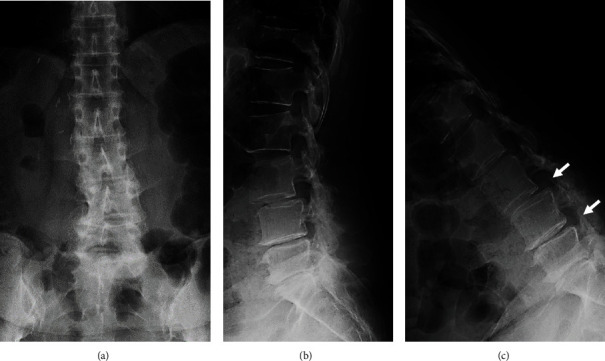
Preoperative lumbar spinal radiographs of a 49-year-old woman with degenerative lumbar disease. (a) anteroposterior view, (b) lateral view, and (c) lateral view (flexion position). Arrows indicate an intervertebral instability at L3/4 and L4/5.
